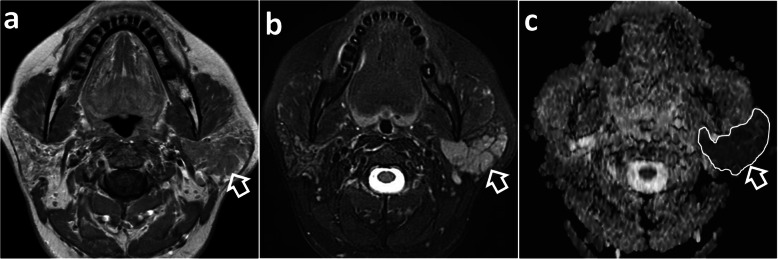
Magnetic resonance imaging of a 40-year-old woman with Sjögren’s disease and left parotid MALToma presenting with long-standing bilateral parotid swelling and 2 years increasing swelling on the left side. a Axial T1-weighted post-contrast image shows a moderately enhancing, homogenous lobulated left parotid mass (open arrow). Both parotid glands exhibit background changes of Sjögren’s disease. b Axial T2-weighted image with fat saturation shows a left parotid mass with intermediate signal intensity (open arrow). Both parotid glands exhibit background changes of Sjögren’s disease. c Axial apparent diffusion coefficient (ADC) map shows markedly restricted diffusion (open arrow) with a very low ADC value of 0.64 × 10^− 3^ mm^2^/s (white contour)
